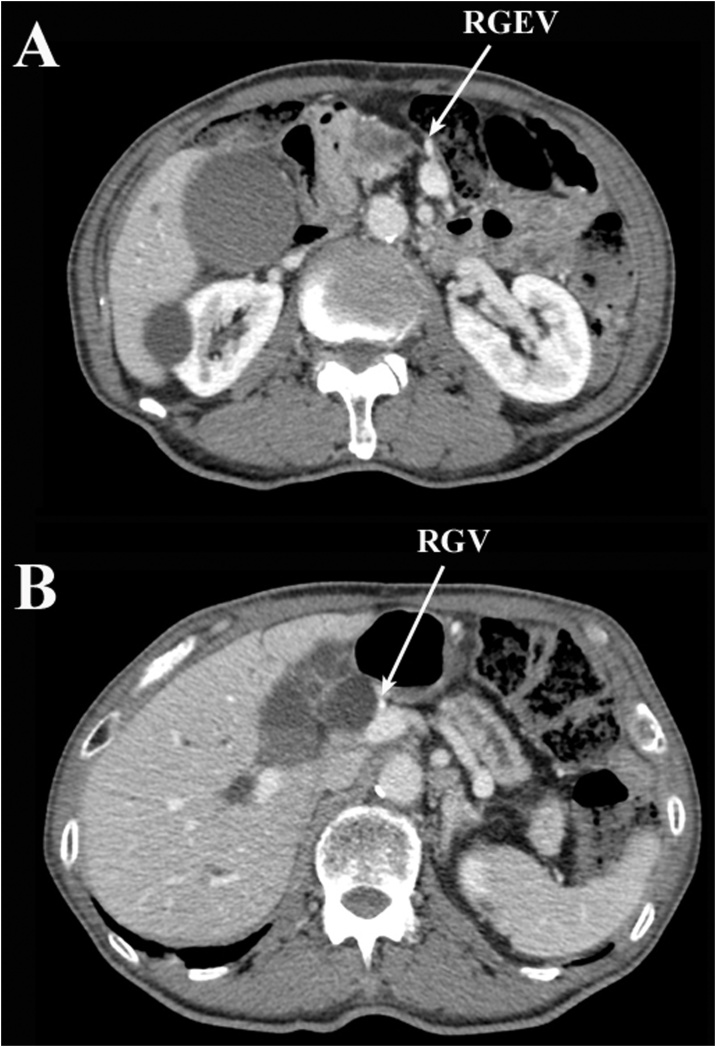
Scans of delayed-phase preoperative computed tomography with contrast medium; A and B: the right gastroepiploic and right gastric veins are patent and act as drainage veins for the gastric tube.
